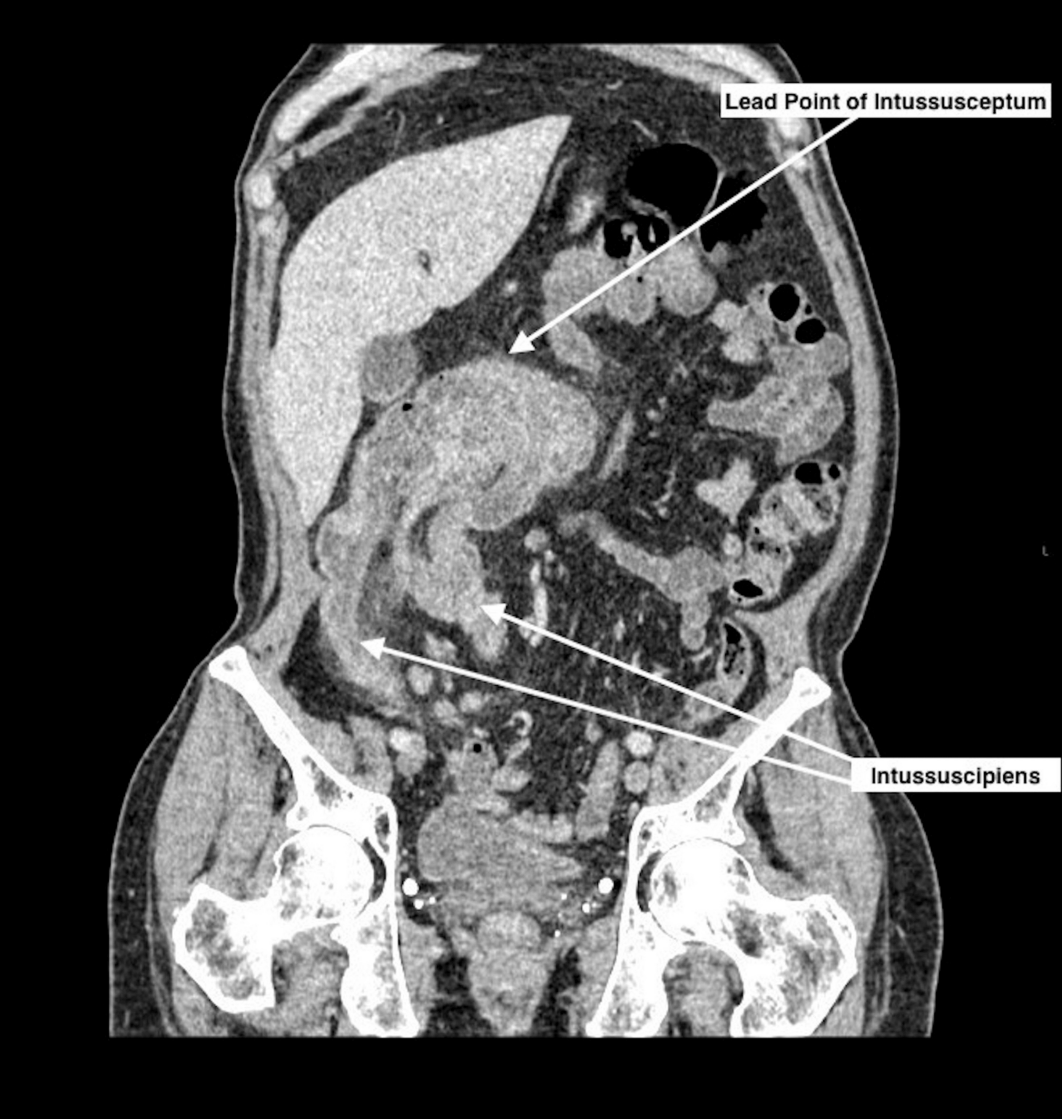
Coronal oblique reconstruction of CT abdomen and pelvis with contrast showing the intussusception. Coronal oblique CT image displaying the bulky lead point of the intussusceptum invaginated into the intussuscipiens.
